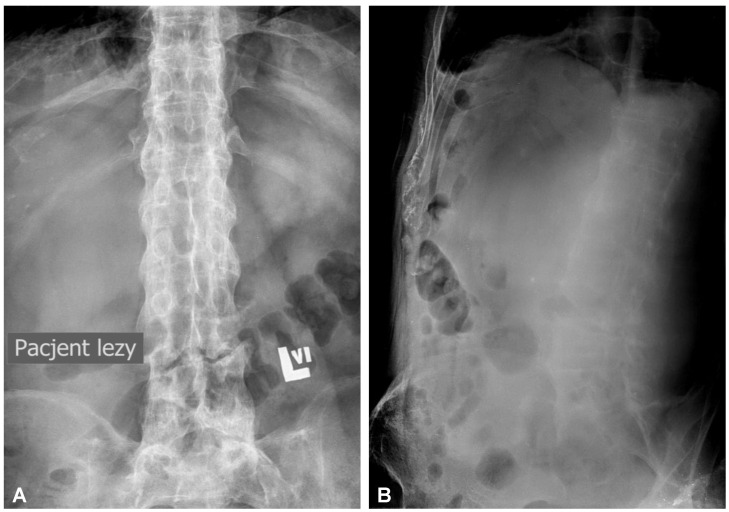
Initial anteroposterior (A) and lateral (B) plain radiographs of the lumbar spine demonstrating fracture line completely slicing through the L3/L4 intervertebral disc space and features of the extensive calcification and ossification along the vertebral column resembling classic “bamboo spine” formation.
